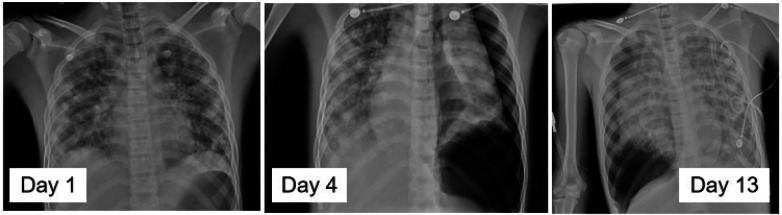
Radiographic findings of the patient's pneumothorax.
